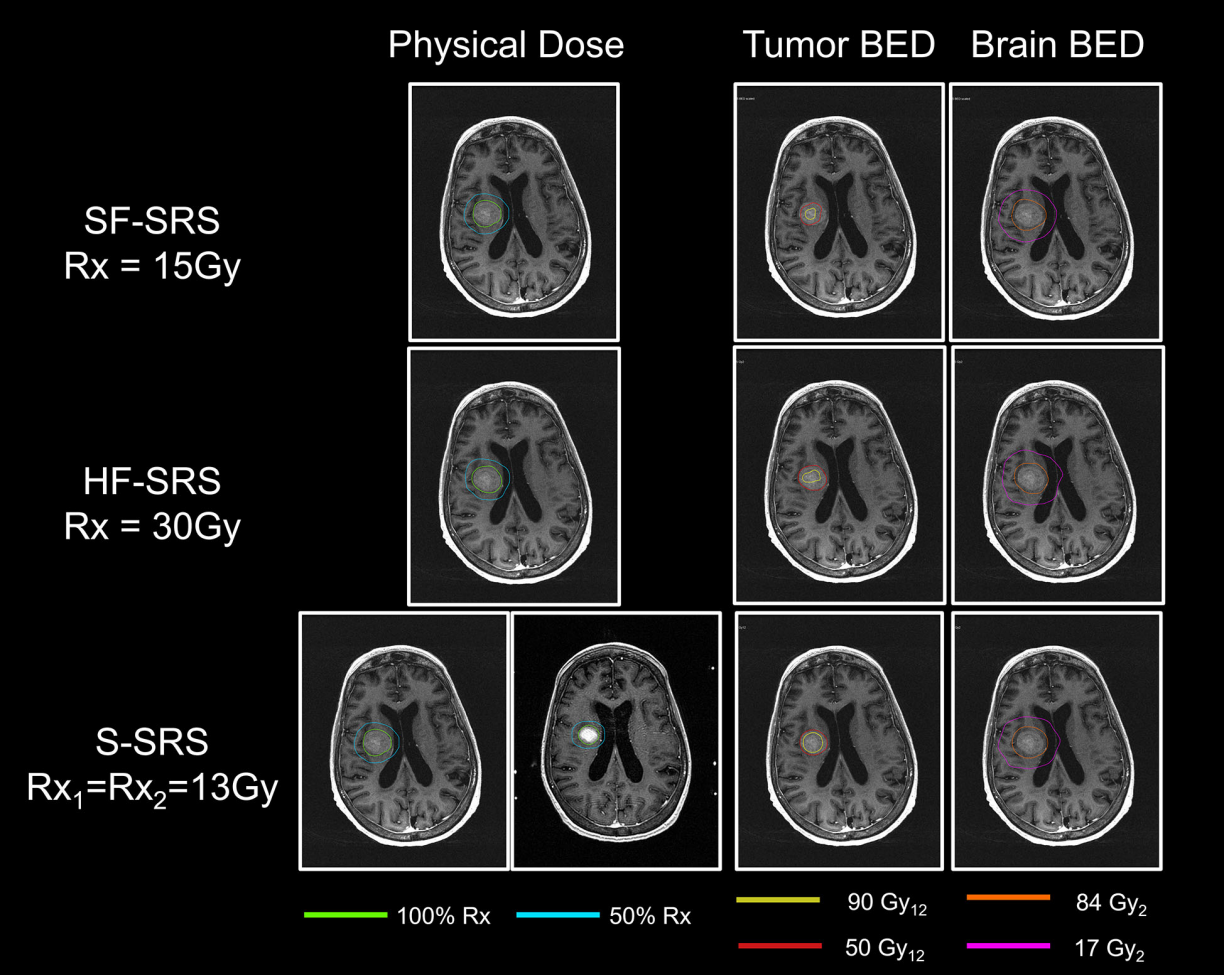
Comparisons of physical dose, total BED to tumor, and total BED to normal brain tissue between SF-SRS, HF-SRS, and Staged-SRS plans for a representative case. The isodose line of 100% and 50% of the prescription dose was shown. The total BEDs were displayed on the MRI acquired in the first session.
